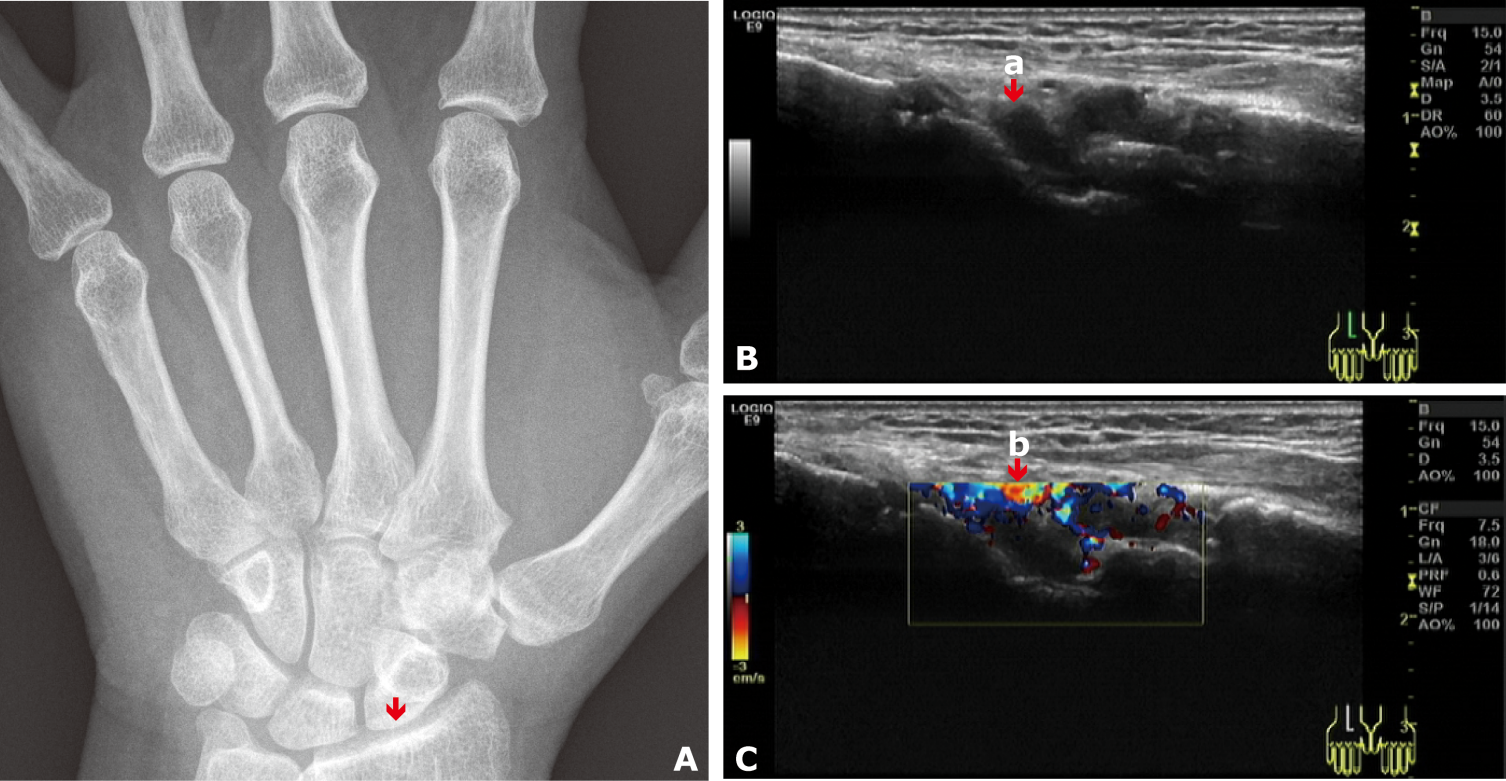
(A) DR image of the left wrist joint showed no signs of RA. The arrow indicates the left radiocarpal joint, where signs were observed on the Doppler scan. (B) Grayscale Doppler sagittal image of the left radiocarpal joint: The arrow (a) indicates synovial hypertrophy. (C) Color Doppler image of the left radiocarpal joint: The arrow (b) indicates hypervascularity.
